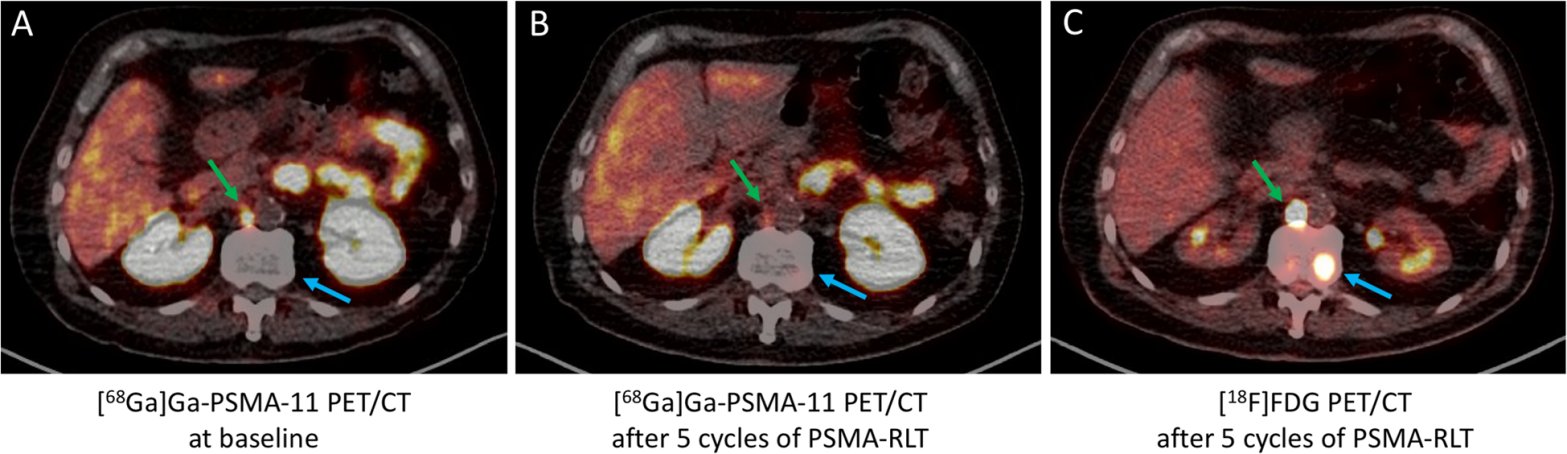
Example of two mismatch lesions detected after 5 cycles of PSMA-RLT: one intensely PSMA-positive (green arrow) and one not identifiable (blue arrow) lesion on the initial [^68^Ga]Ga-PSMA-11 PET/CT at baseline prior to PSMA-RLT (a). [^68^Ga]Ga-PSMA-11 PET/CT (b) and [^18^F]FDG PET/CT (c) after 5 cycles of PSMA-RLT showing [^18^F]FDG/[^68^Ga]Ga-PSMA-11 mismatch in both lesions
